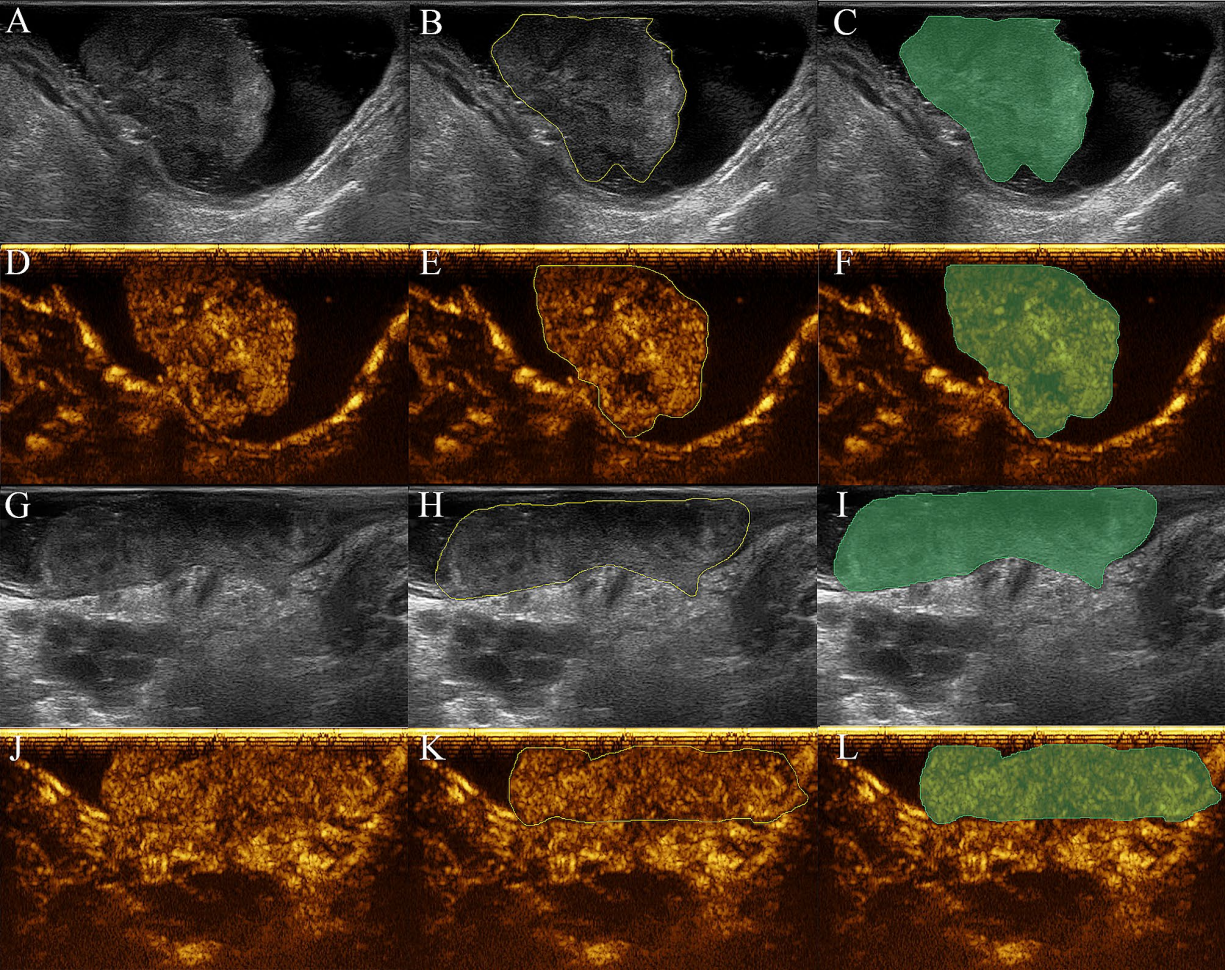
Region of Interest (ROI) for rectal cancer tumors. (A-F) A patient who had a good response after neoadjuvant chemoradiotherapy. (G-L) A patient who had a poor response after neoadjuvant chemoradiotherapy. A, G Raw images of tumors from B-mode ultrasound; D, J Raw images of tumors from contrast-enhanced ultrasound. B, E, H, K tracing tumors’ ROI (yellow curve represents the tracing pathway); C, F, I, L Confirming tumors’ ROI (green area)
